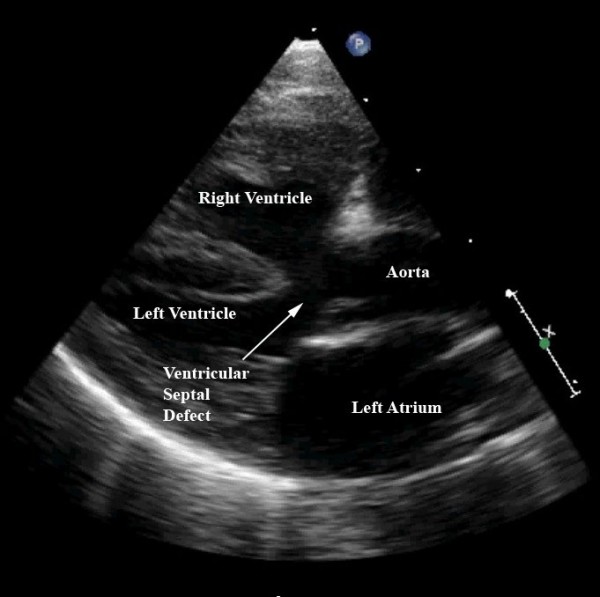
This still frame of a modified parasternal long axis view from the same patient as imaged for Figures 6 and 7 demonstrates the large ventricular septal defect, aortic override, and right ventricular hypertrophy charactistic of patients with tetralogy of Fallot.
